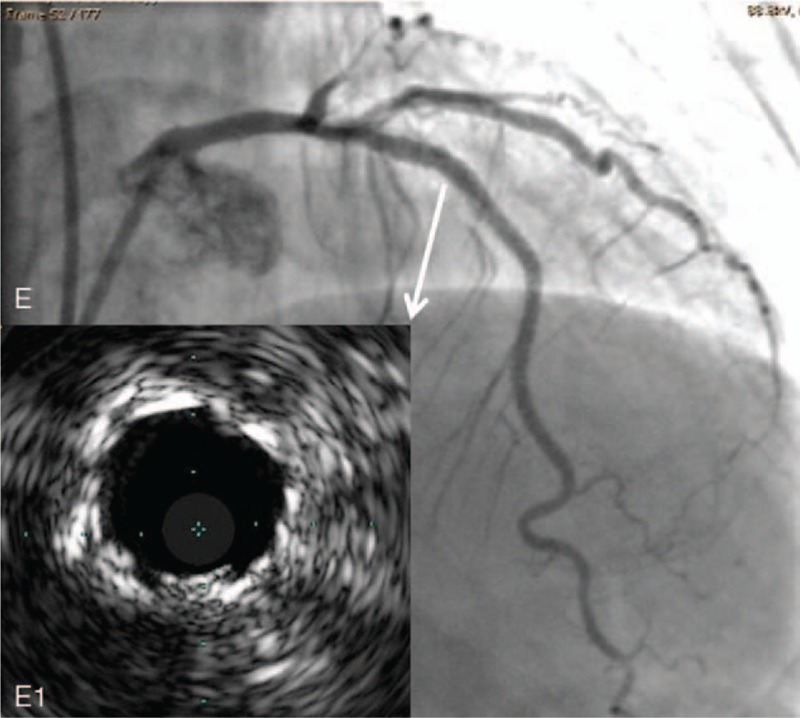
Final result. (E) The final angiogram showed fully expanded LAD stent. (E1) IVUS showed the bilayers stent in the proximal LAD (white arrow), and the final lumen area was 7.2 mm^2^. LAD = left anterior descending artery, IUVS = intravascular ultrasound.
